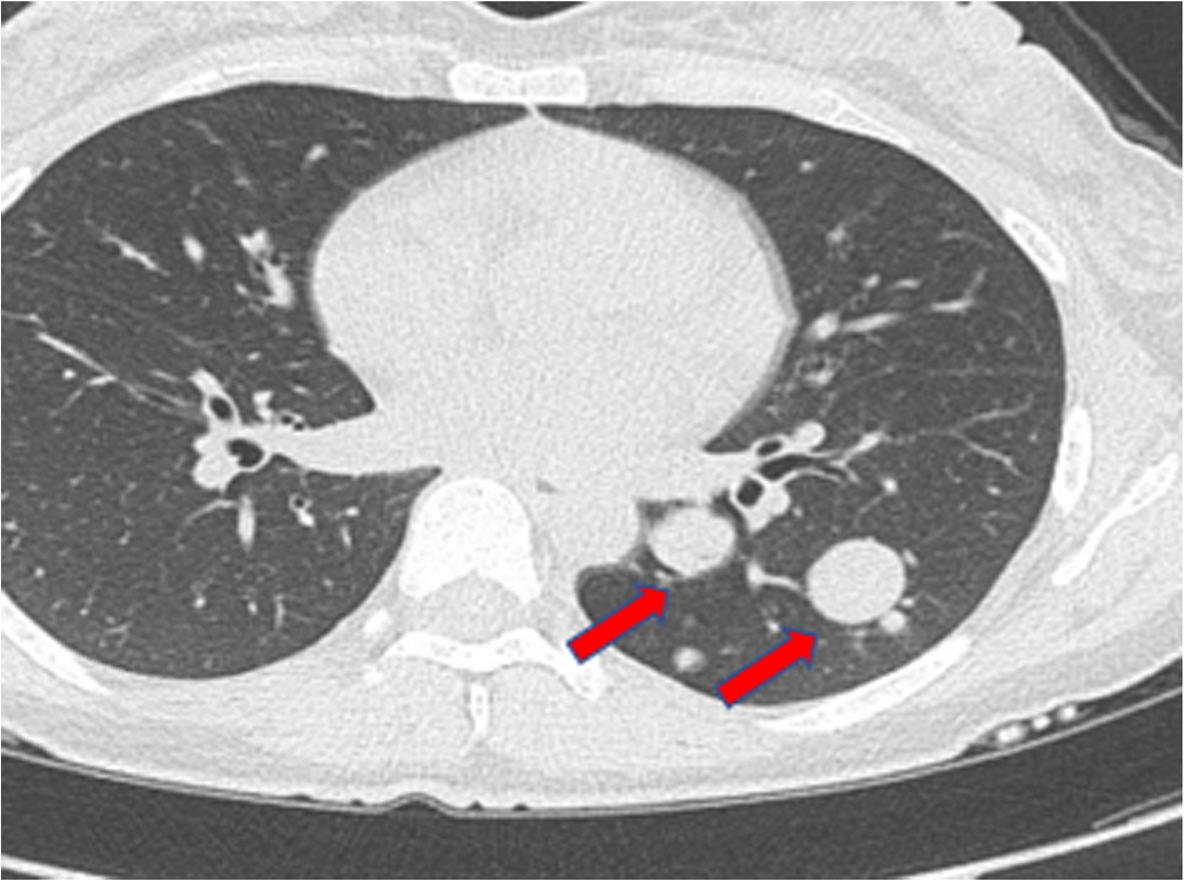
Chest CT showed multiple (approximately 49) nodules and a maximal 2.8 cm nodule in diameter in the left lower lobe
